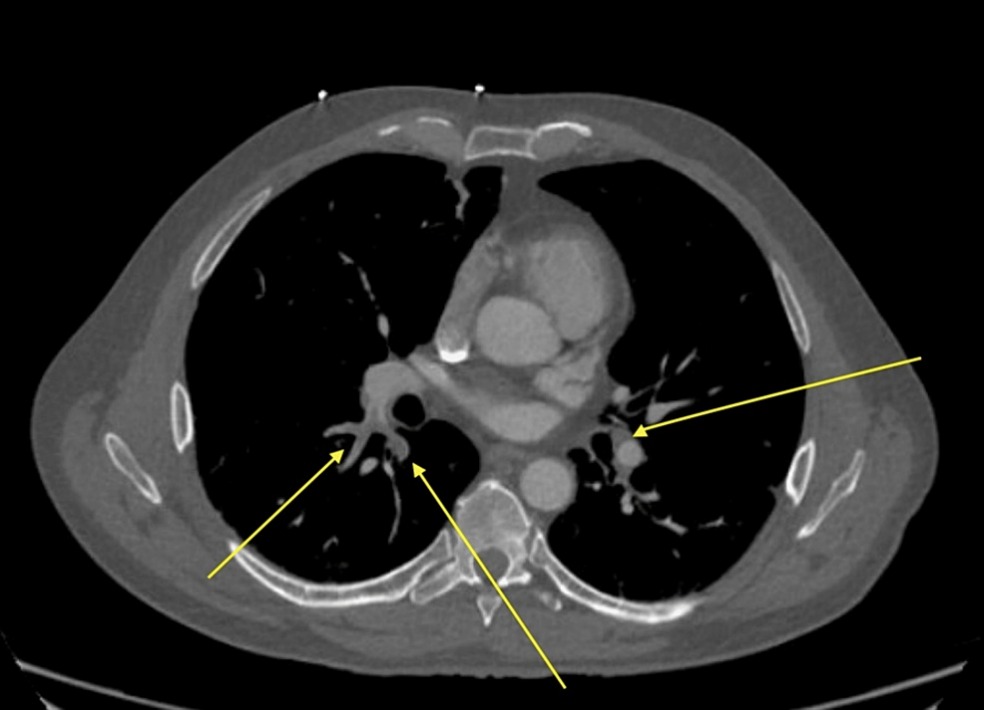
Day one, CT chest angiogram revealed pulmonary embolism within the segmental and sub-segmental branches of the right lung and sub-segmental branches of the left upper and right middle lobes (yellow arrows)
